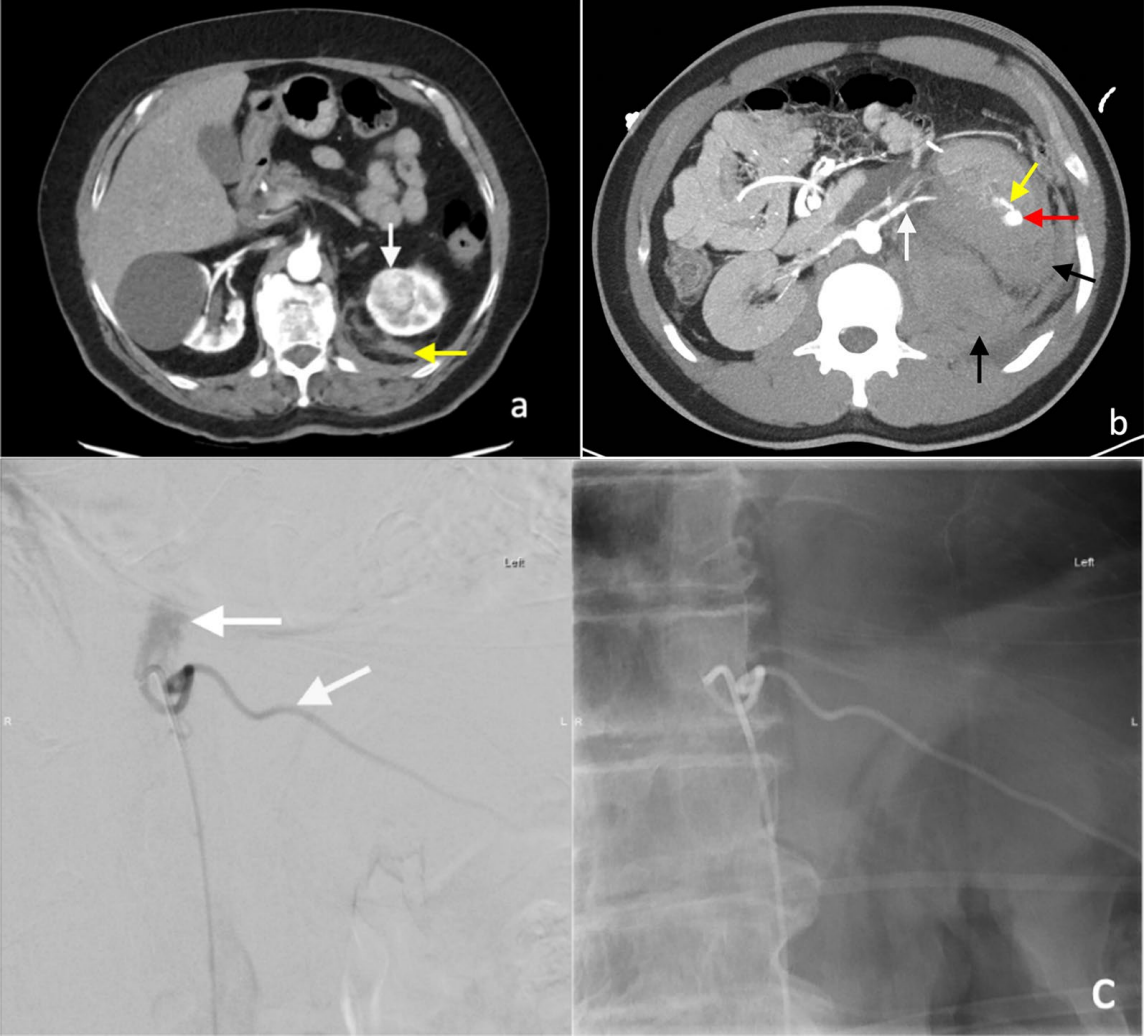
a Axial contrast-enhanced arterial phase CT image following a CT-guided 18-gauge core biopsy of a left upper pole renal tumour (white arrow) with adjacent perinephric haematoma and stranding (yellow arrow). b A more superior arterial phase axial CT image in the same patient demonstrated active extravasation of contrast (white arrow) within the small left haemothorax (yellow arrow) from a left intercostal artery at T11 (red arrow). c Active contrast extravasation (white arrow) from a left T11 intercostal artery (yellow arrow) was confirmed on angiography and successfully treated with embolisation coils
